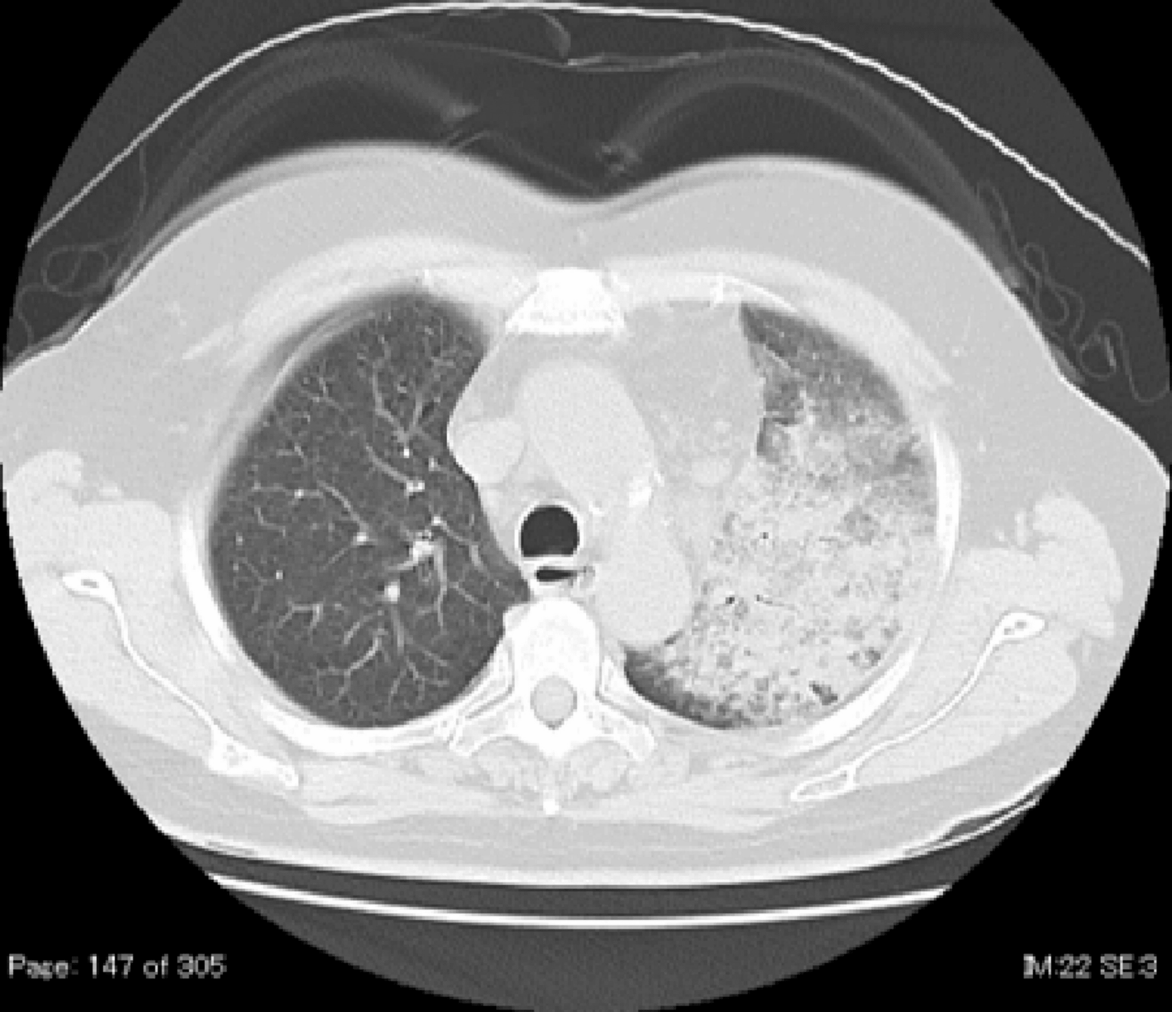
Plain CT of the chest on admission. Plain CT revealed an infiltrating shadow in the left lung.
